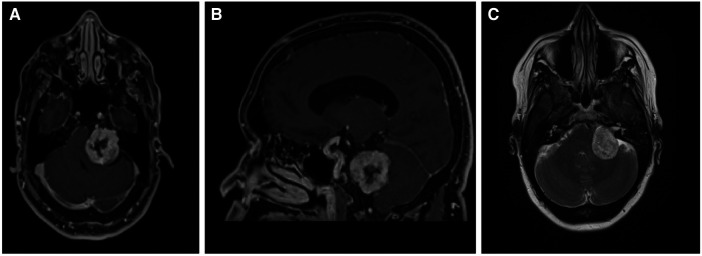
Diagnostic MRI study demonstrating the vestibular schwannoma (A) axial T1 with contrast; (B) sagittal T1 with contrast (C) axial T2.
